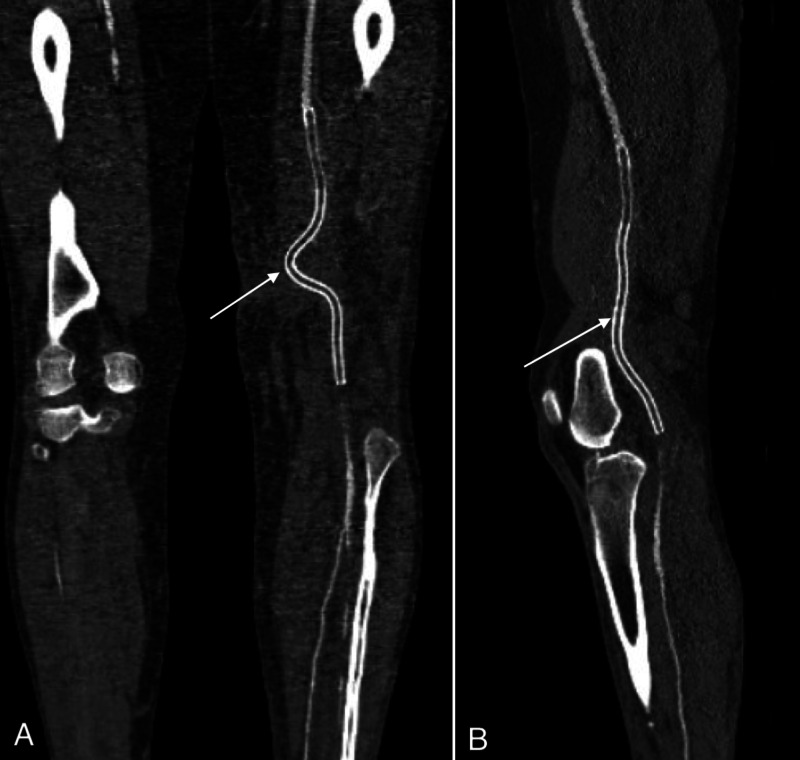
(A, B) Control CTA shows occluded and cranially retracted stent and stent-grafts (arrow). CTA - Computed tomography angiography
